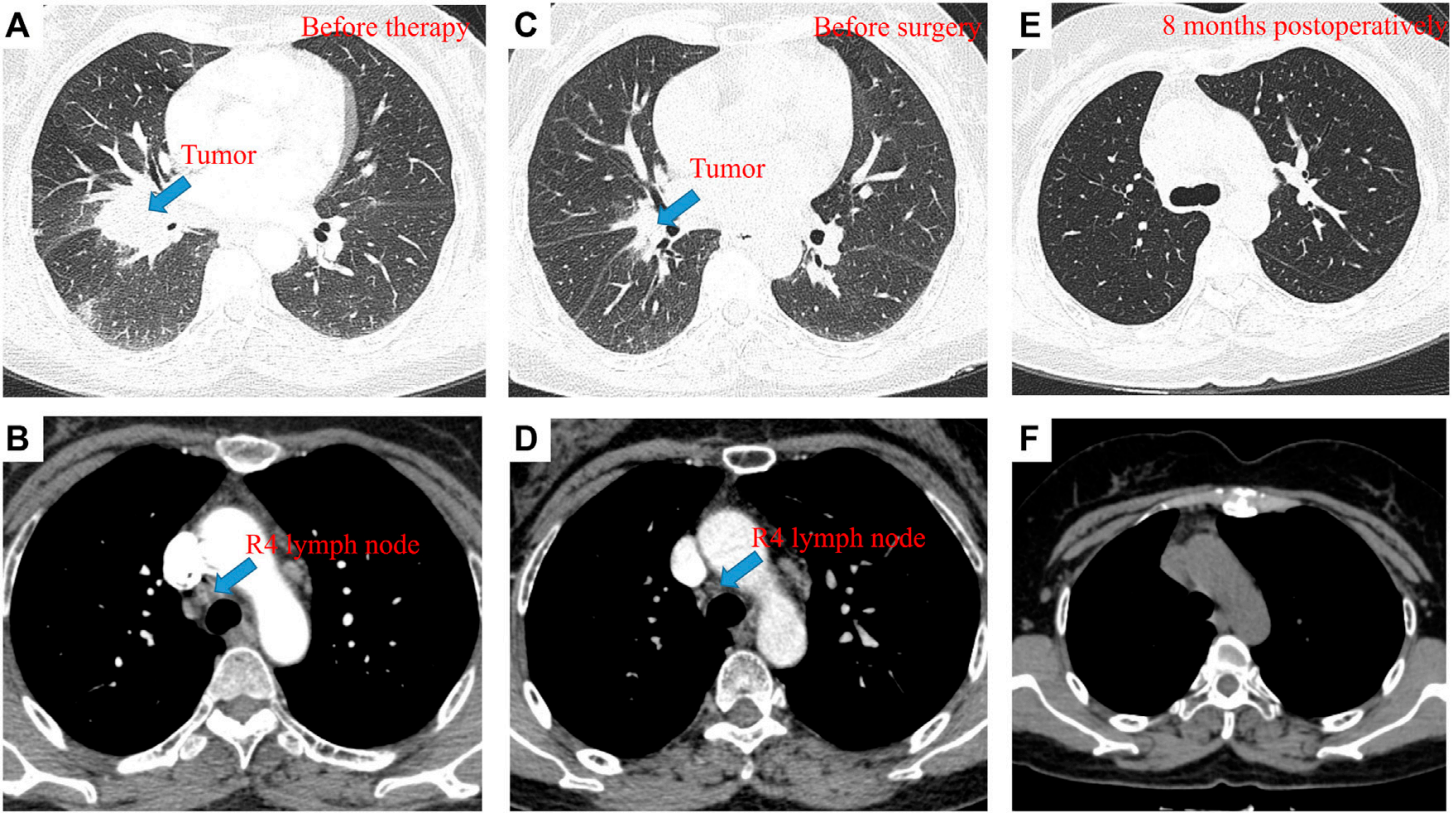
pCR to neoadjuvant alectinib in resectable ALK-positive NSCLC. Contrast-enhanced CT of the chest before neoadjuvant therapy showed an approximately 4.2 × 3.4 cm mass in the right hilum with localized obstructive pneumonia in the right lower lobe (A) and enlarged R4 lymph node (B); Contrast-enhanced CT of the chest after neoadjuvant therapy showed an approximately 1.2 × 1.1 cm mass in the right hilum, with a tumor shrinkage of 90% (C) and 0.6 × 0.6 cm R4 lymph node, with a shrinkage of 50% (D); Contrast-enhanced CT of the chest at the 8-month follow-up showed no local replase (E,F).
